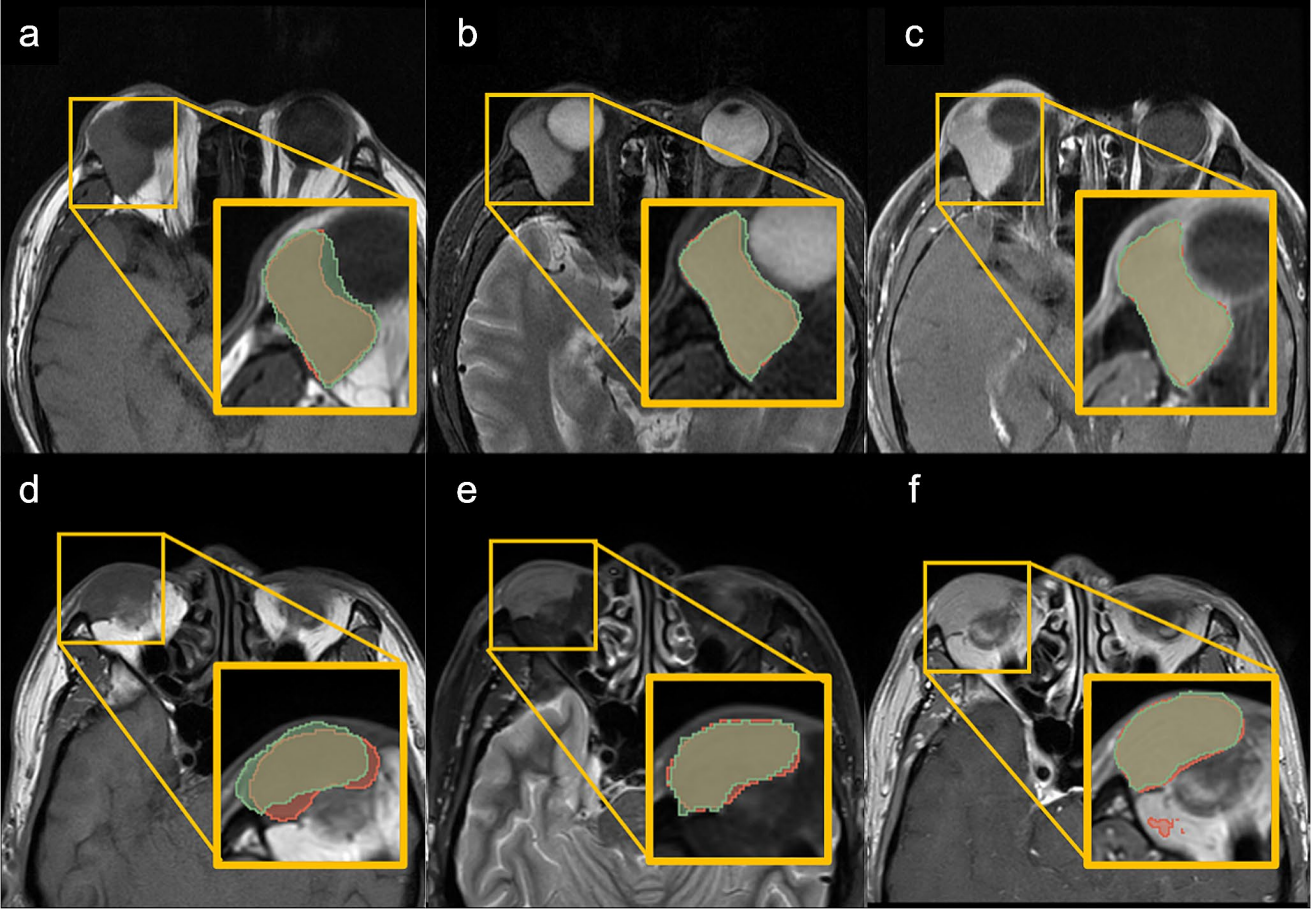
Tumor segmentation performance of OAL with multi-sequence MRI images in Model 1. In the labeled images, the green area represents the manual segmentation, and the red area represents the predicted tumor by nnU-net. a-c A 75-year-old male with OAL of the right lacrimal gland. The DSCs of T1 (a), T2_FS (b), and T1c_FS (c) are 0.89, 0.90, and 0.95, respectively. d-f A 46-year-old male was diagnosed with OAL of the right superior outer orbit. The DSCs of T1 (d), T2_FS (e), and T1c_nFS (f) are 0.78, 0.84, and 0.89, respectively
